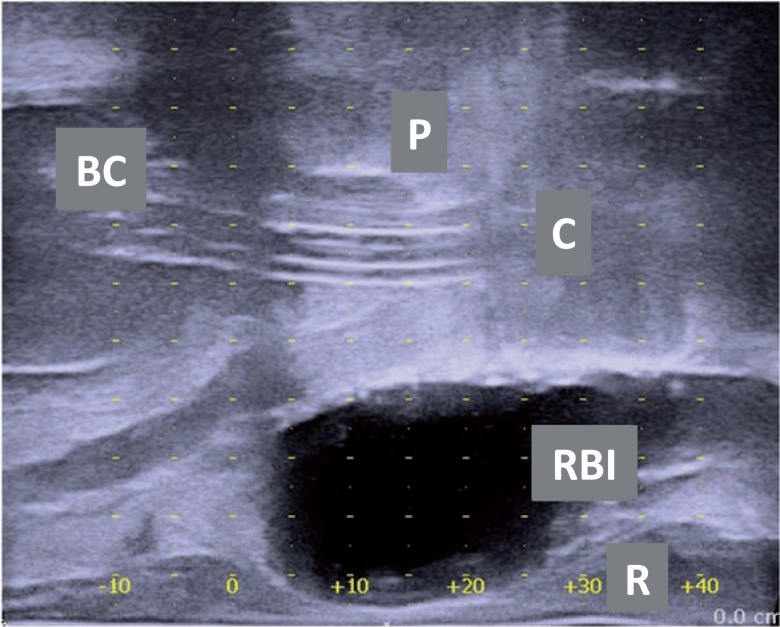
Sagittal ultrasound image of patient with a rectum balloon implant (RBI) *in situ* between the prostate (p) and the rectum (R). The view is in the central plane with the urinary catheter (C) and the foley balloon catheter (Bc) visible in the bladder.
